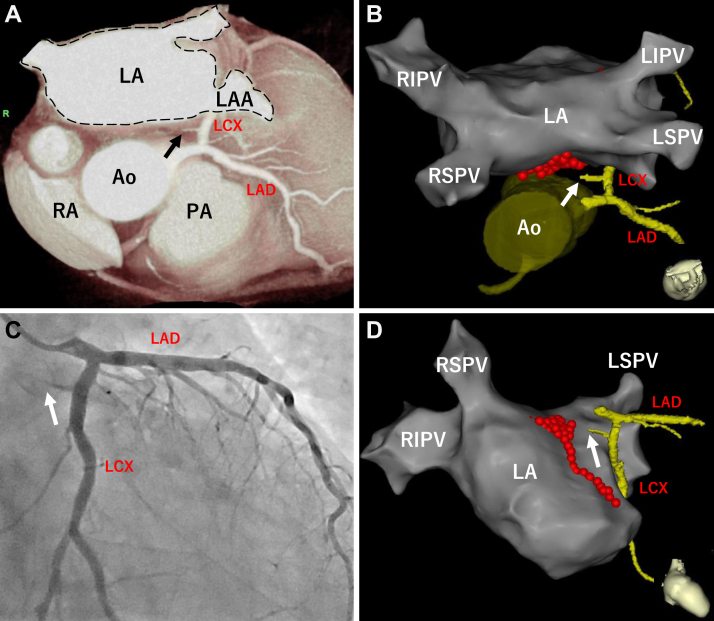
The contrast between coronary computed tomographic angiography (CCTA) or coronary angiography and the 3-dimensional map of the left atrium (LA). A: Volume-rendered view of CCTA showing occlusion of the sinus nodal artery (*black arrow*) originating from the left circumflex artery (LCX). B: The fusion of CCTA showing the left coronary artery and the anatomical shell of the LA is presented. The sinus nodal artery (*white arrow*) was occluded near the ablation point of the left atrial anterior wall (*red tag*). C: Coronary angiography showed an occluded sinus nodal artery (*white arrow*) originating from the LCX. D: The fusion of CCTA showing the left coronary artery and the anatomical shell of the LA is presented. The sinus nodal artery (*white arrow*) was occluded near the ablation point of the left atrial anterior wall (*red tag*). Ao = aorta; LAA = left atrial appendage; LAD = left anterior descending artery; LIPV = left inferior pulmonary vein; LSPV = left superior pulmonary vein; PA = pulmonary artery; RA = right atrium; RIPV = right inferior pulmonary vein; RSPV = right superior pulmonary vein.
